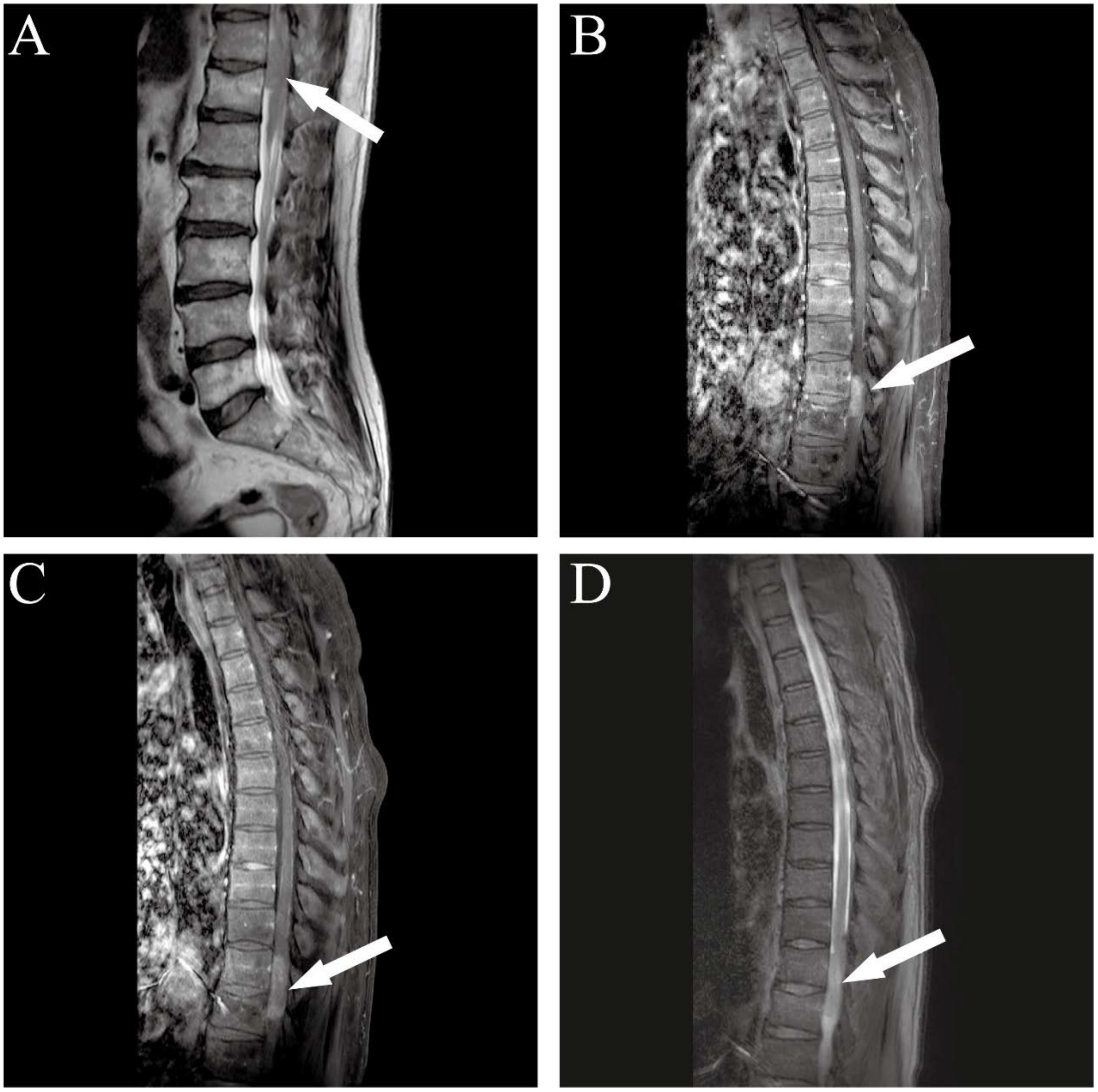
Sagittal MRI of the thoracic and lumbar spine. (A) T2-weighted imaging (T2WI) without contrast demonstrates an extramedullary subdural lesion at the T11–T12 spinal canal level (white arrow), exhibiting iso- to mildly hyperintense signal relative to the spinal cord. (B) Contrast-enhanced T1-weighted imaging (T1WI+C) reveals homogeneous enhancement of the lesion with well-defined margins, consistent with an extramedullary subdural tumor. (C) Follow-up T1WI+C demonstrates an increase in lesion size compared to baseline, accompanied by mildly prolonged T2 signal intensity within the spinal cord at levels T10–T12. (D) Corresponding fat-suppressed T2-weighted imaging (T2-FS) confirms a hyperintense intramedullary area from T10 to T12, correlating with findings on (C), with effective suppression of surrounding fat signal.
